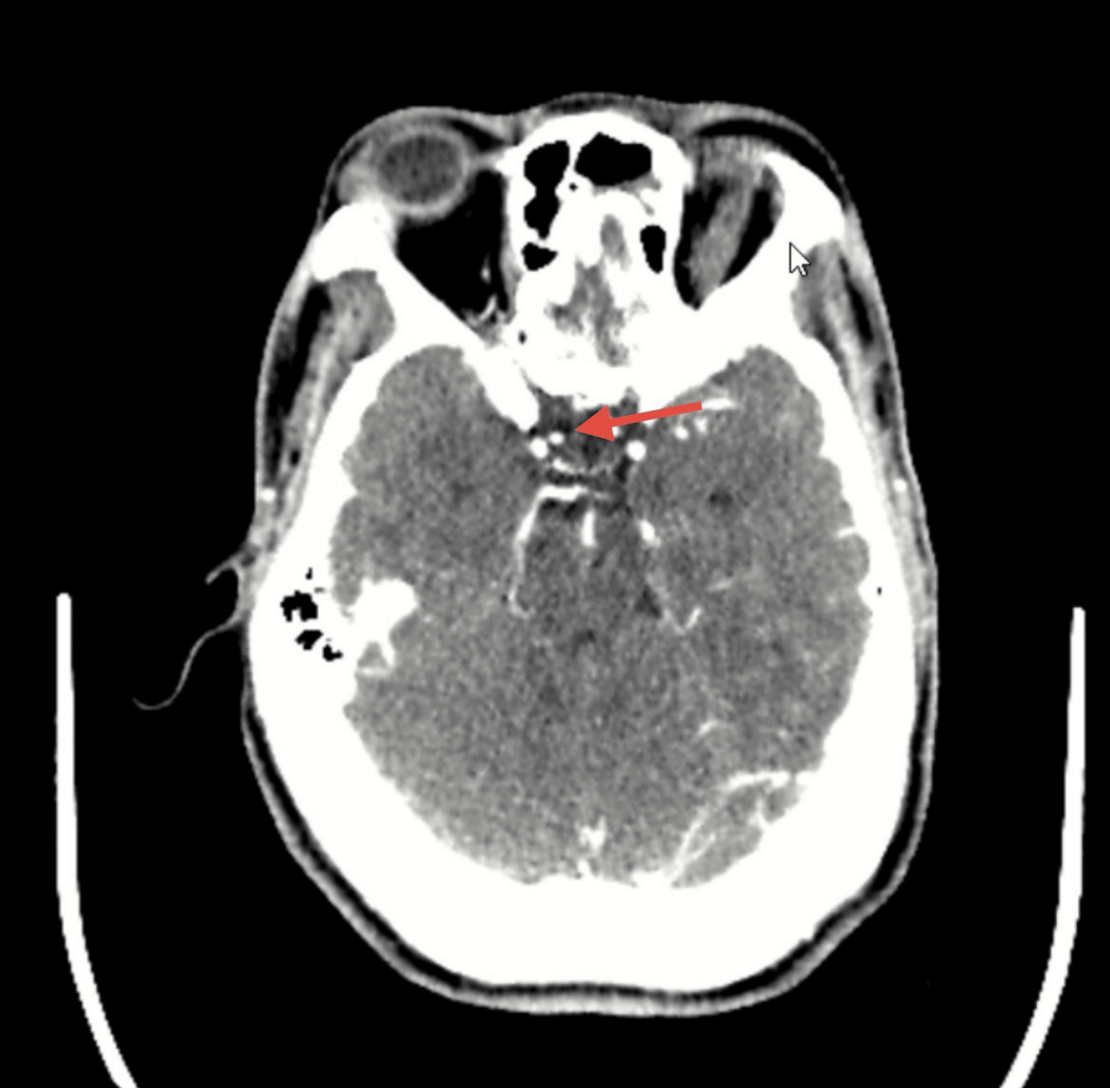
Contrast-enhanced CT angiography Axial angiographic image showing a saccular aneurysm in the clinoid segment of the right internal carotid artery (red arrow), without signs of rupture.
